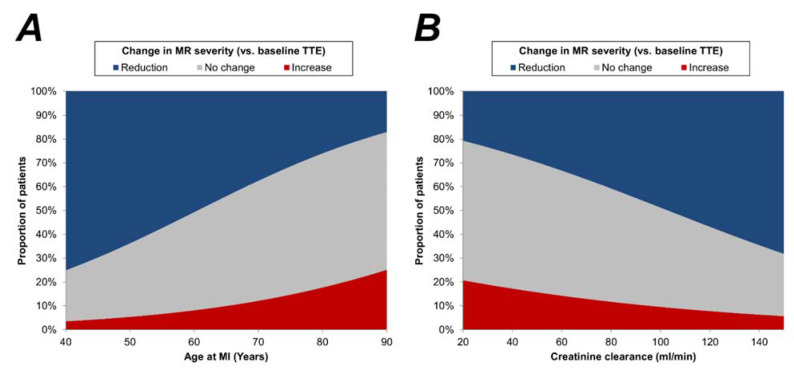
Associations between age/creatinine clearance and changes in MR severity. Plots are based on binary logistic regression models, with either the age (A) or creatinine clearance (B) as a continuous covariate. Two models were produced for each factor, with the dependent variable being reduction in MR severity (vs. increase or no change) in the first, and increase in MR severity (vs. reduction or no change) in the second. For all models, the changes in MR severity were assessed between the baseline and final TTE for each patient. The two models were then evaluated to estimate the rates of reductions and increases in MR severity for each value of the covariate, with the difference between these assumed to be the rate of “no change”. MI = myocardial infarction; MR = mitral regurgitation; TTE = transthoracic echocardiography.
